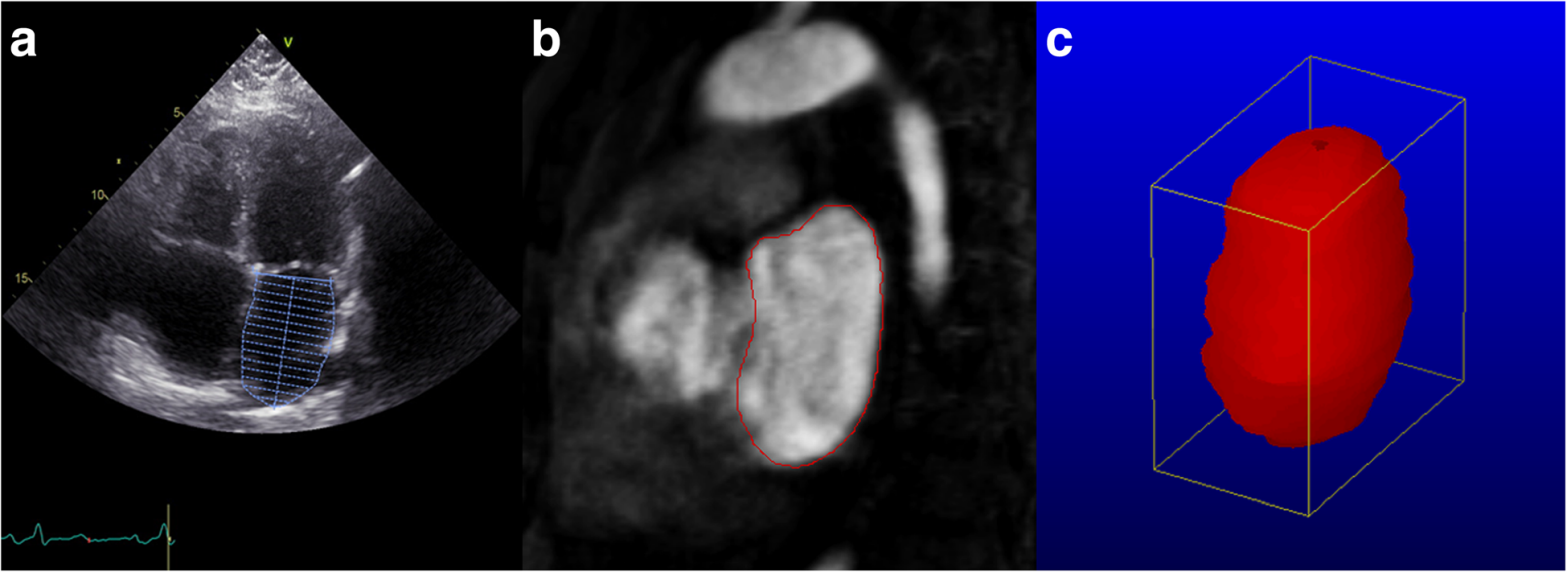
LA volume assessment with TTE and CE-MRA. a Example of a TTE apical 4-chamber view with delineation of the LA wall and exclusion of the LAA, PVs and area between the mitral valve annulus and mitral valve leaflets. Landmarks indicate the mitral valve level and LA length. b Example of a sagittal segment of CE-MRA with delineation of the LA wall. The atrioventricular groove and mitral valve were used as landmarks to separate the LA from the left ventricle. c 3-D reconstruction of the LA cavity, constructed from the CE-MRA segments
